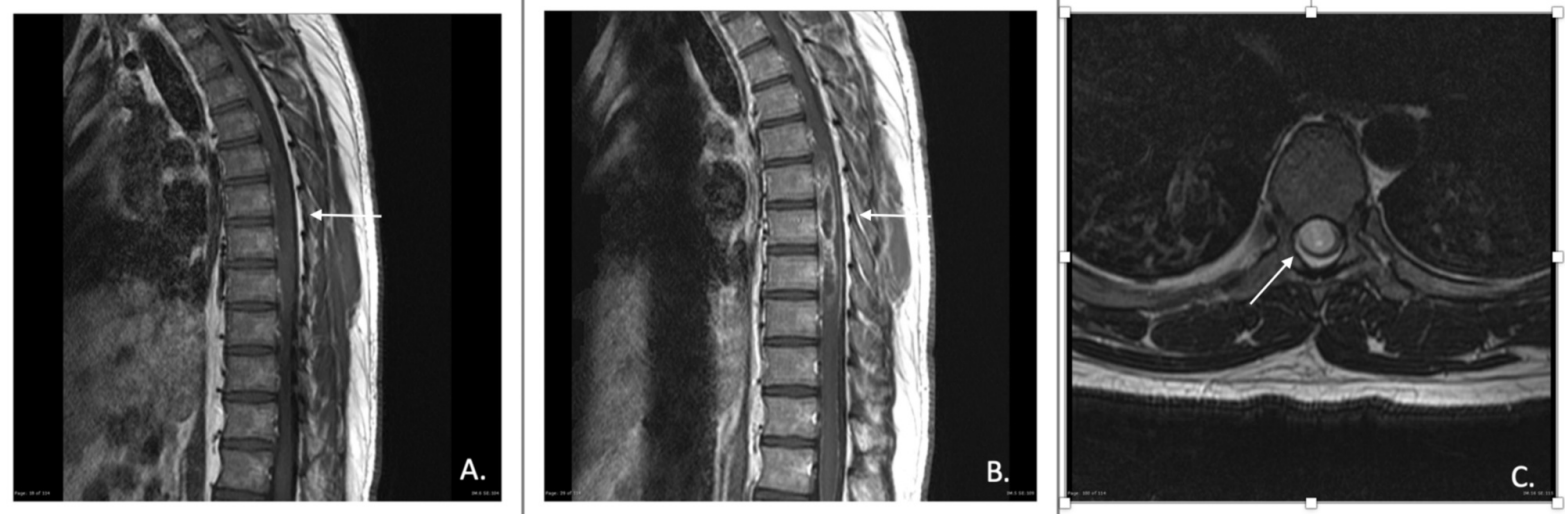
High-grade astrocytoma of the thoracic spine. A) T1-weighted sagittal pre-contrast image, B) T1-weighted sagittal post-contrast image, and C) T2-weighted axial slice showing displacement of the thoracic cord by area of hyperintensity consistent with spinal astrocytoma. Photo Reference: Courtesy of Assoc. Prof. Frank Gaillard, Radiopaedia.org, rID: 19536
